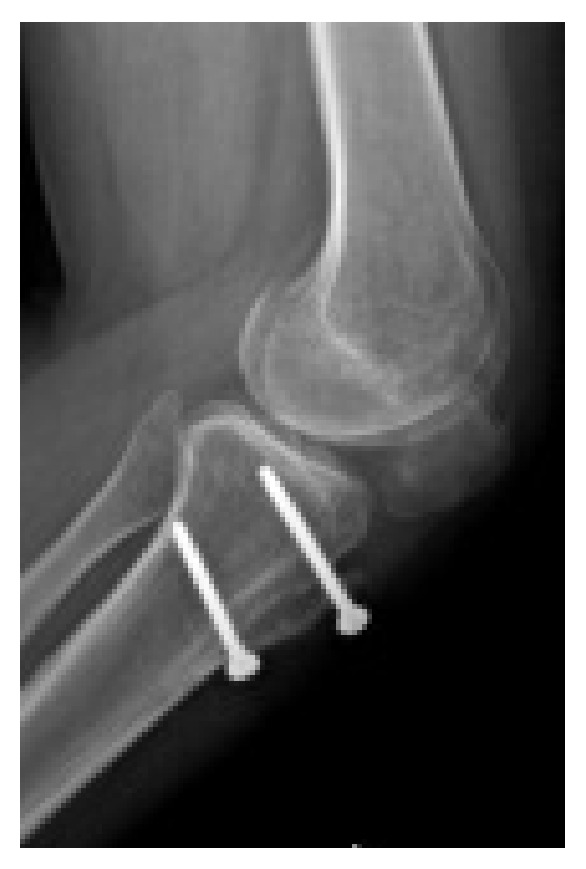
Pre-op lateral knee X-ray.
